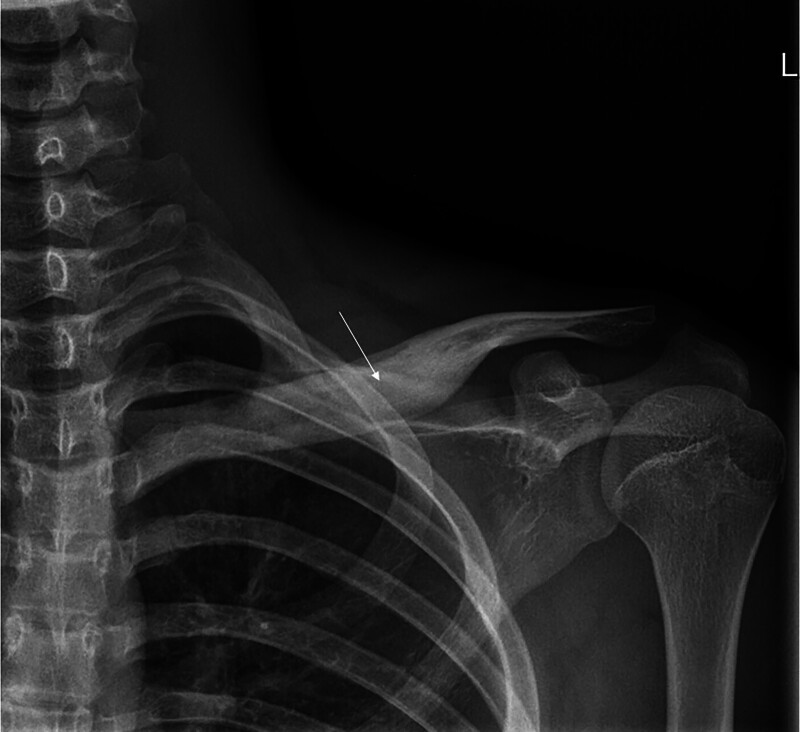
Radiograph showed areas of osteosclerosis (white arrow) on the clavicle of a 12-year-old boy.
